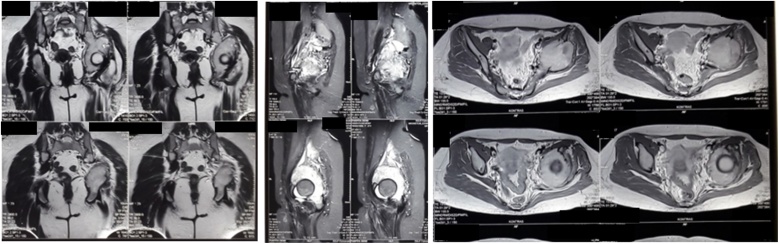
MRI Examination of the Pelvis.
